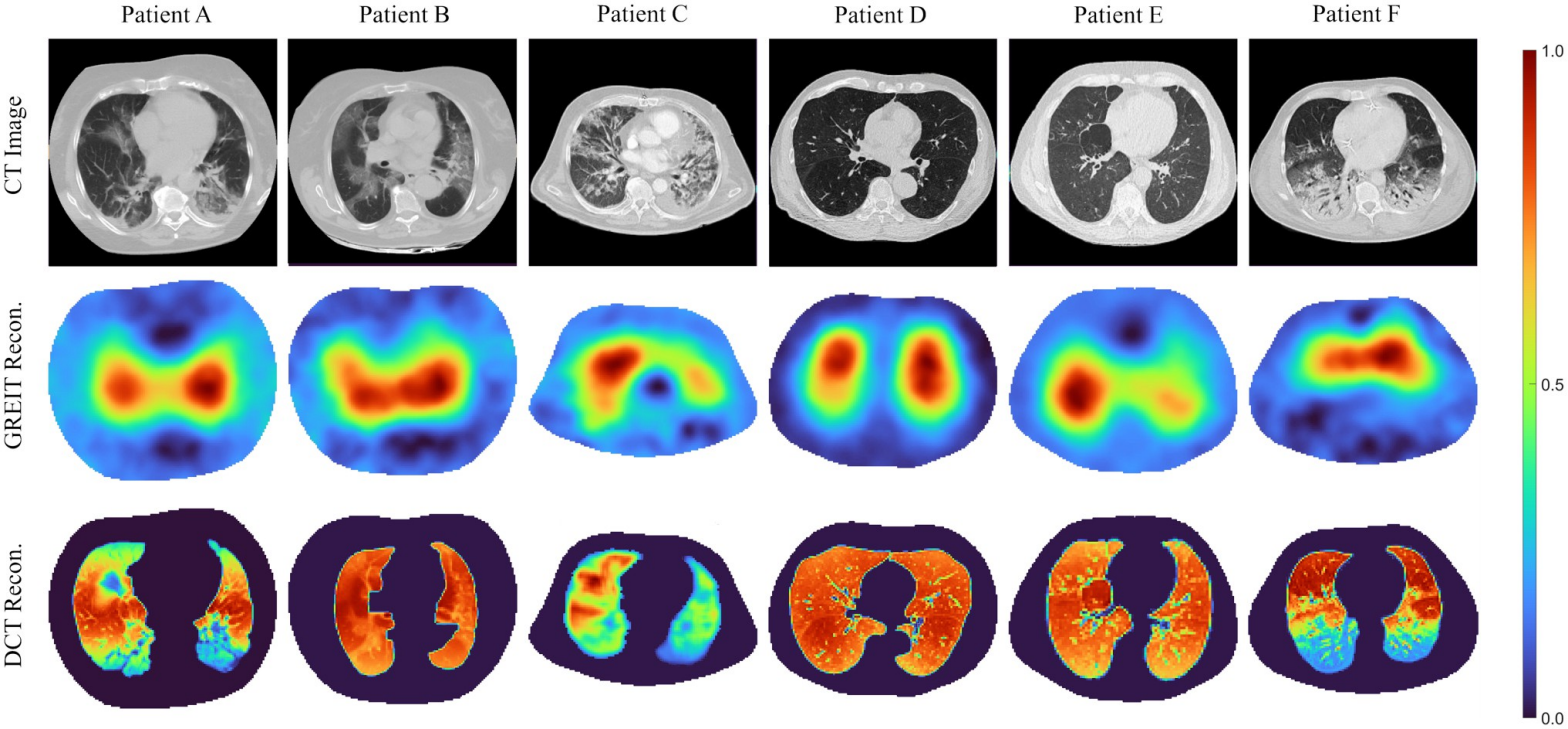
First row: CT images of the patient, which are used for the segmentation of the lung and thorax shape for EIT reconstruction. Second row: GREIT reconstructions of conductivity change. Third row: Reconstructions of conductivity change using the DCT-based approach with detail prior derived from the related CT image.
